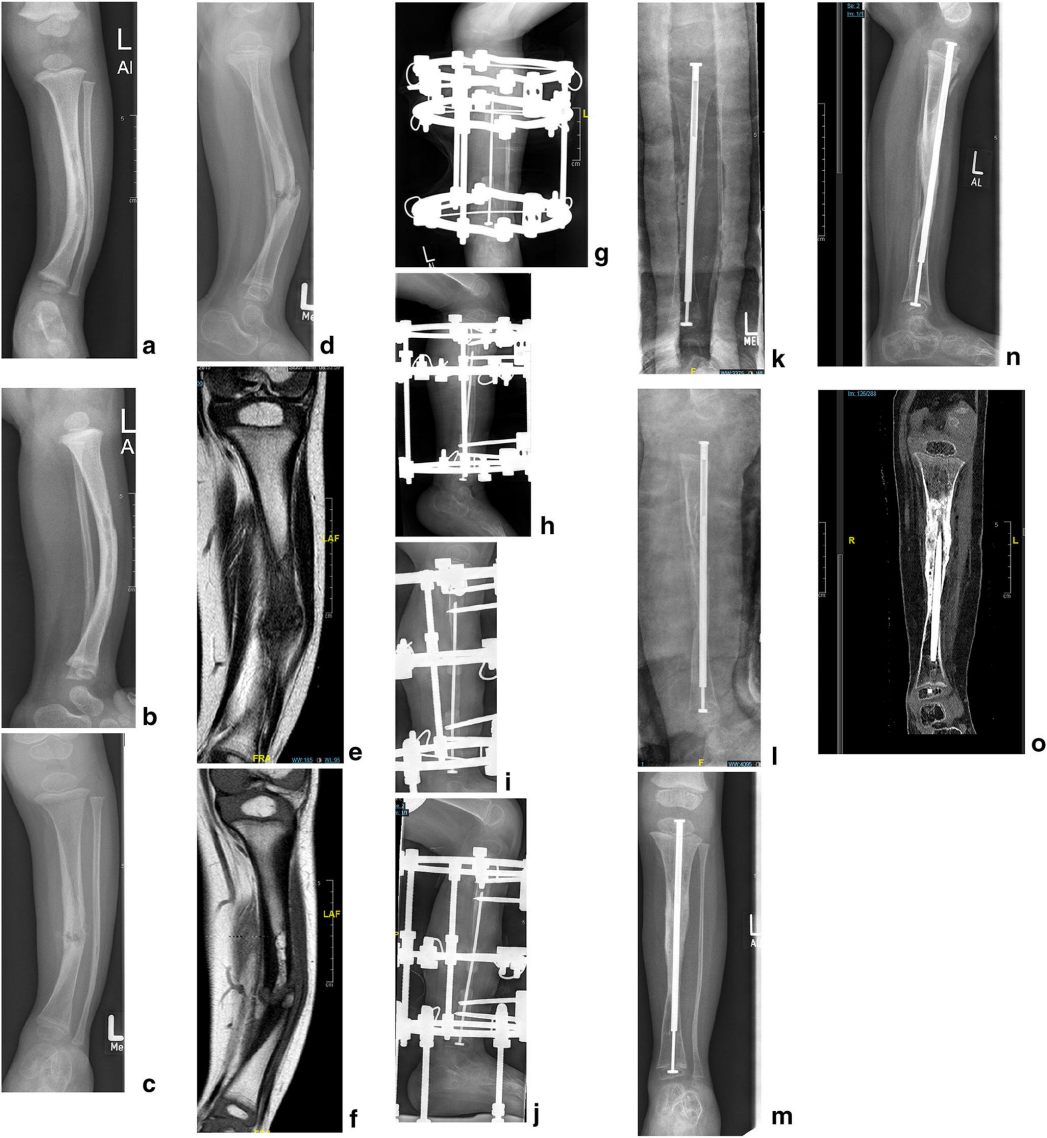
(Case 9) Antero-lateral tibial bowing (a–o) in a 3-year-old child (a, b). Pathological fracture at age 4.5 years despite bracing (c, d). The extent of dystrophic changes is seen on MRI (e, f). Resection of the pseudarthrosis, retrograde intramedullary nailing and application of a ring fixator for segment bone transport in order to fill the defect (g, h). Bone segment transport with concurrent distraction osteogenesis(i, j). Six weeks after the “Docking procedure” after completion of the bone segment transport with telescopic intramedullary nail osteosynthesis and rhBMP-2 application. The external ring fixator is already removed (k, l). Final result with solid union a.p. (m), lateral (n), CT scan (o). The intramedullary telescopic nail is retained, the child should wear a protective “double shell” brace until maturity.
